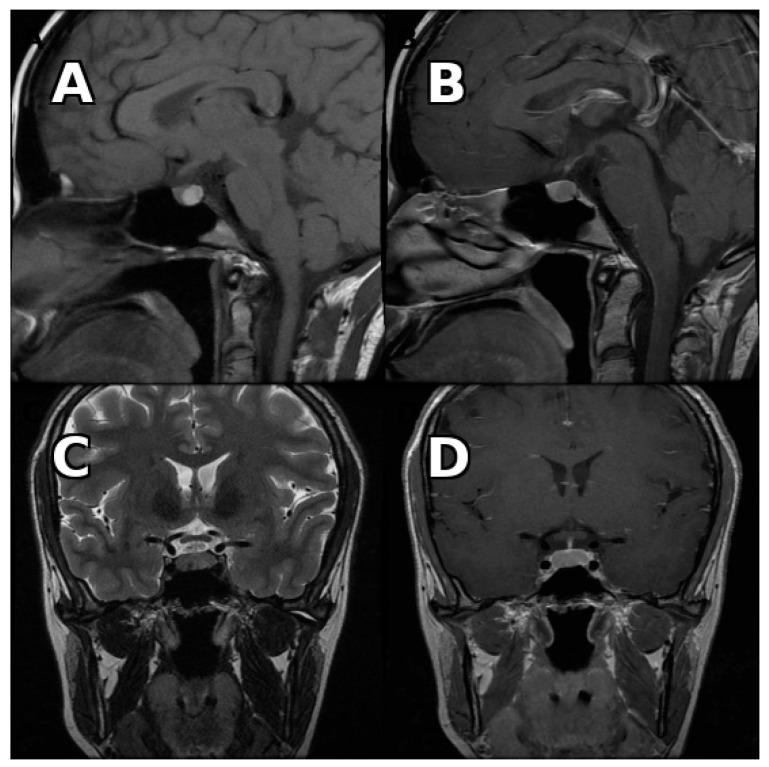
Pituitary MRI of a patient with Rathke’s cleft cyst. (A) Sagittal T1-weighted image demonstrating spontaneous hyperintensity of the cystic lesion. (B) Contrast-enhanced sagittal T1-weighted image demonstrating no enhancement. (C) Coronal T2-weighted image demonstrating midline localization. (D) Contrast-enhanced coronal T1-weighted image demonstrating the midline position without enhancement.
